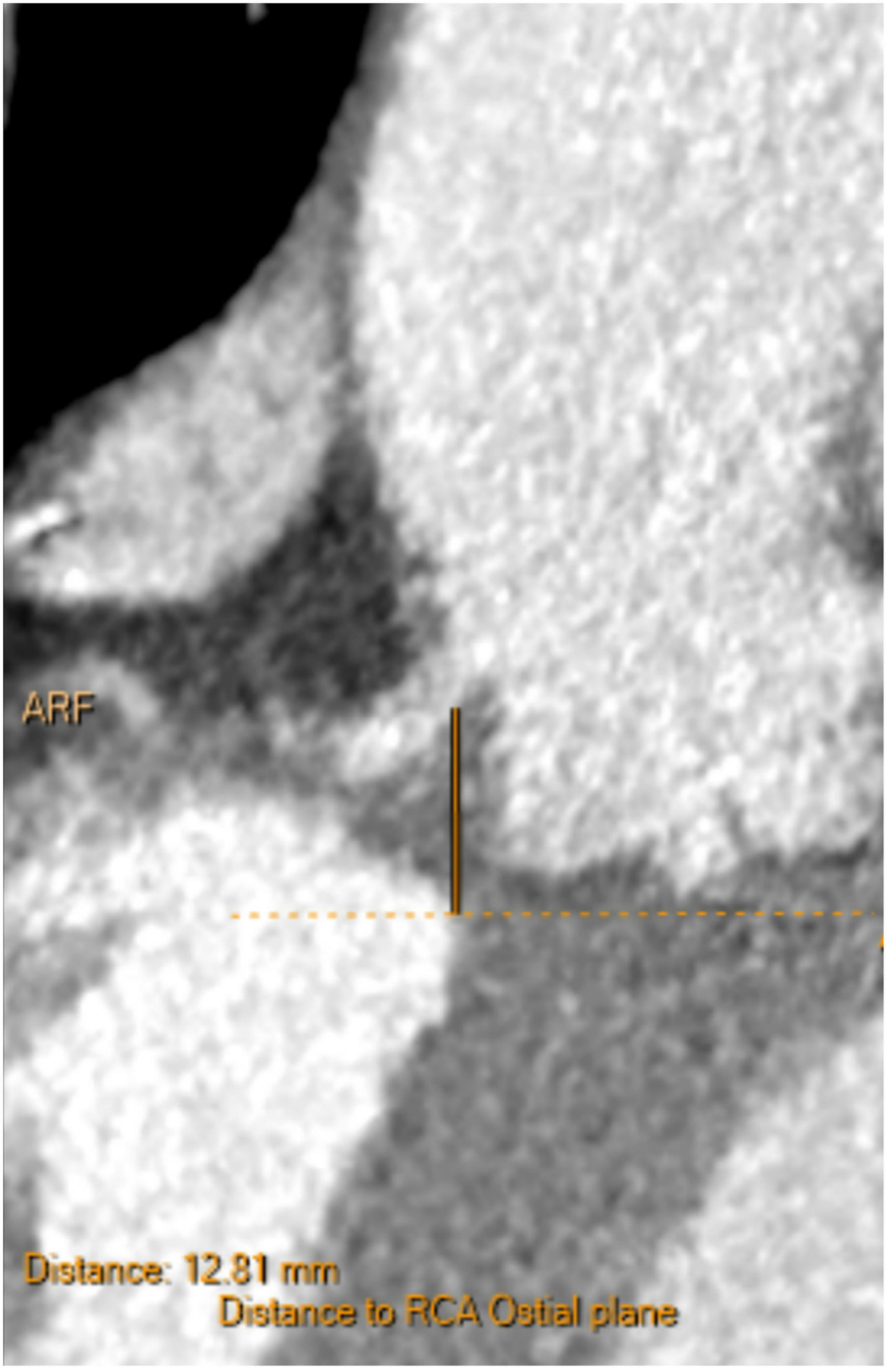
Pre-procedural CT showing the native right coronary ostium 12.8 mm above the annular plane, indicating a safe coronary height prior to valve implantation. No leaflet calcification or sinus sequestration was observed.
